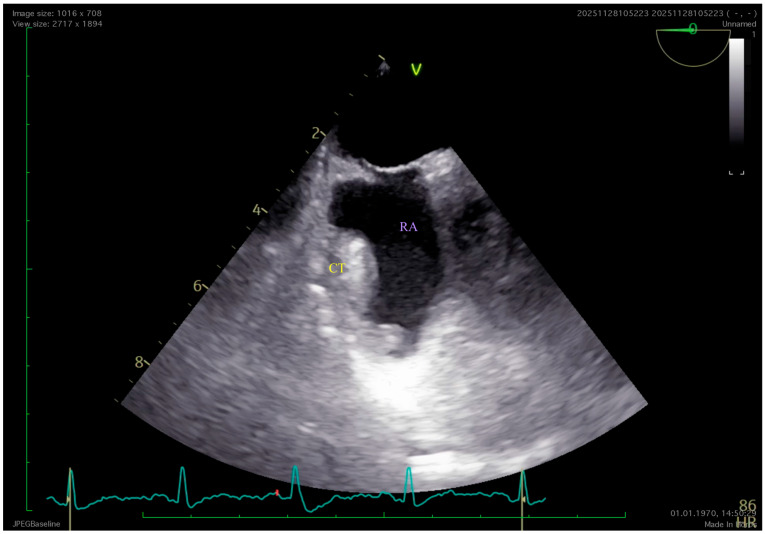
TEE showing a hypertrophied crista terminalis along the lateral right atrial wall; CT: crista terminalis, RA: right atrium.
